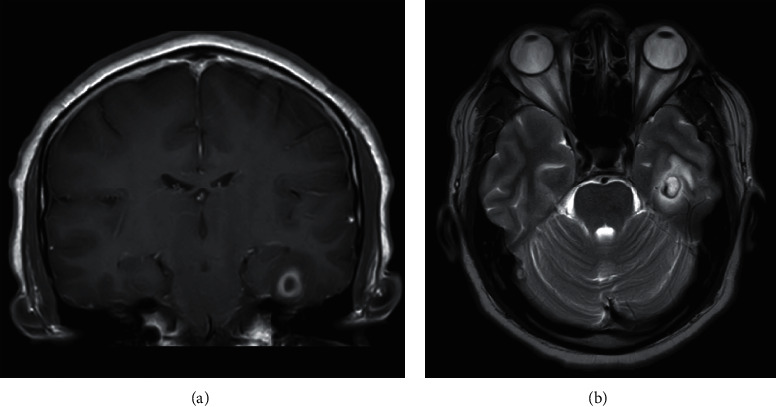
Magnetic resonance images of the left temporal lobe lesion. (a) Contrast-enhanced coronal T1-weighted image, showing rim-enhancing left temporal lobe lesion. (b) Axial T2-weighted image showing the same lesion with mild surrounding vasogenic edema.
